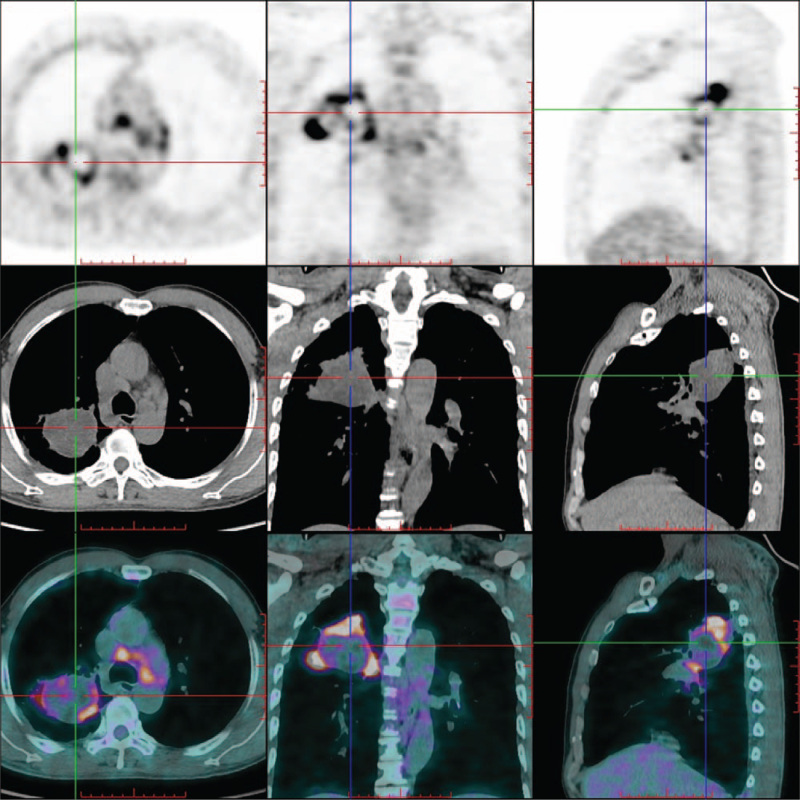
The whole body 3D PET/CT images showed a cystic-solid mass in the right thorax with irregular margins. The thickness of the cyst wall was not uniform, the cystic wall showed significantly increased FDG uptake, but the central cystic component was not accompanied by FDG uptake. Several enlarged lymph nodes with increased FDG uptake could be seen in the mediastinum. (top row: PET scan images; middle row: unenhanced CT scan images; and bottom row: PET and CT fusion images). FDG = fluorodeoxyglucose, PET/CT = positron emission tomography/computed tomography.
